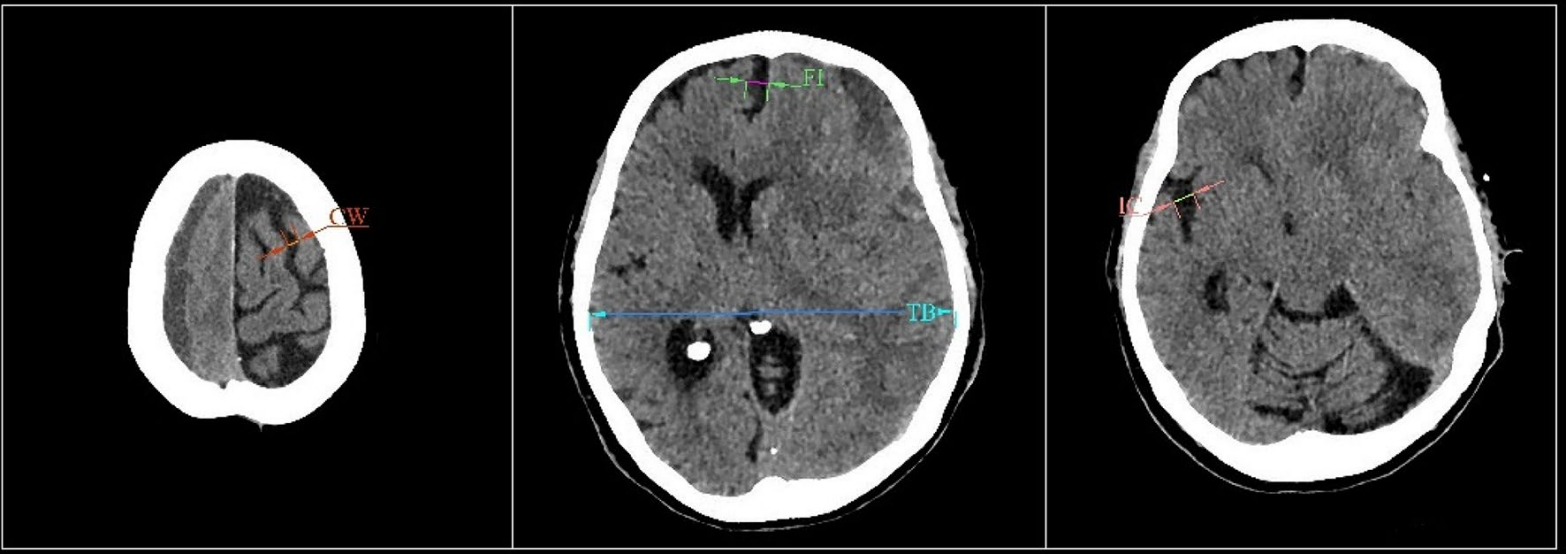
RCA Index is developed on the parameters adopted from Chrzan et al.^1^: the Insular Cisterns width (IC), the longitudinal cerebral fissure width in the anterior part (FI), and the cerebral Sulci greatest Width at the skull vault (SW) in mm in the hemisphere contralateral to the hematoma related to the Temporal Bone greatest internal distance (TB). These parameters are measured on CT scan axial images.
